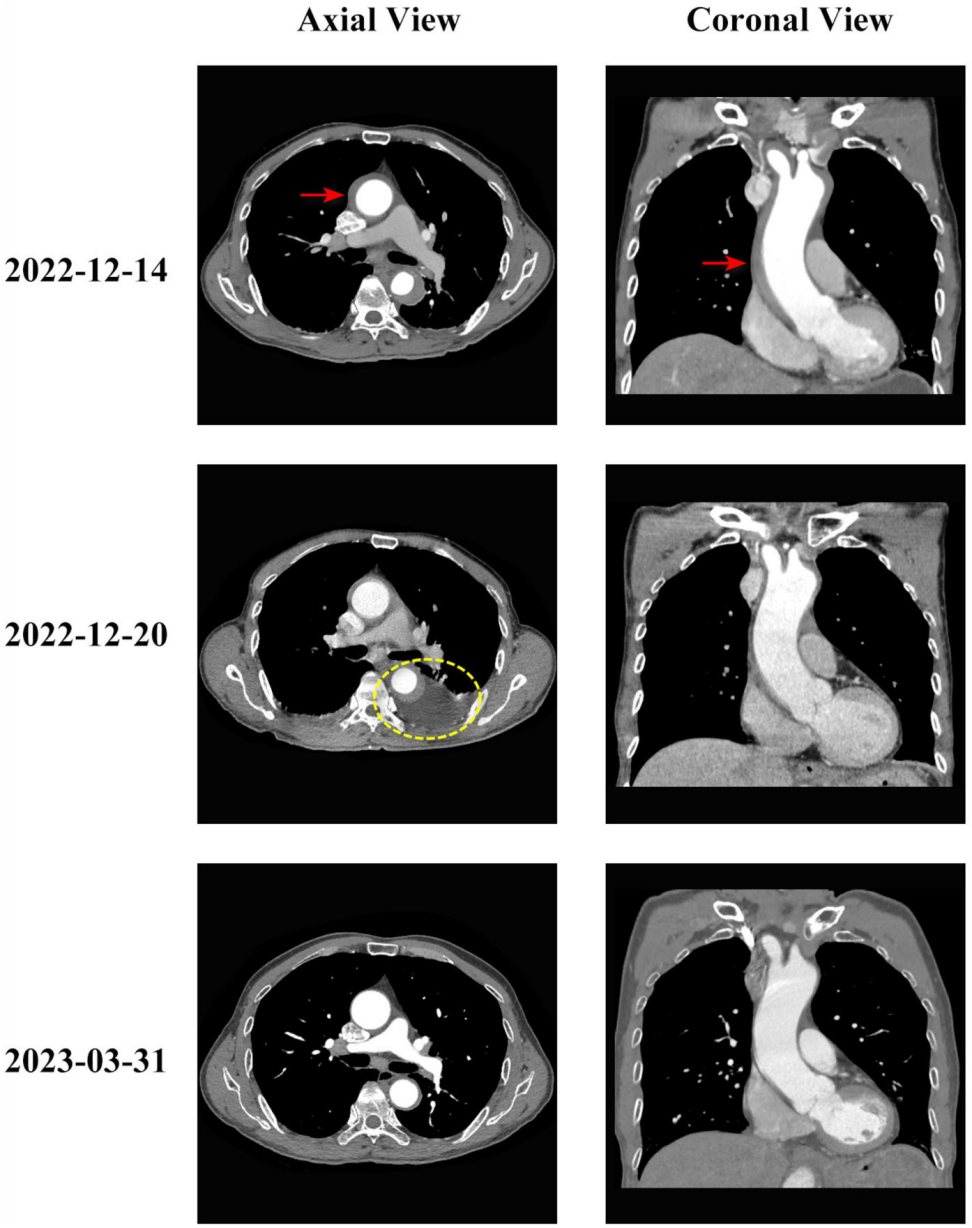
Evolution of type A aortic intramural hematoma during conservative treatment. Serial computed tomography angiography (CTA) images showing the evolution of type A aortic intramural hematoma in axial (left column) and coronal (right column) views. Top row (2022-12-14): Initial presentation showing type A intramural hematoma involving the ascending aorta (red arrows indicate intramural hematoma location) with thickening of the aortic wall (hematoma thickness 7 mm). The ascending aortic diameter measured 42 mm. Middle row (2022-12-20): One weeks after conservative treatment initiation, showing resolution of the ascending aortic intramural hematoma but development of periaortic hematoma around the descending aorta (yellow circle indicates periaortic hematoma). Bottom row (2023-03-31): Three months follow-up demonstrating complete resolution of both the intramural hematoma and periaortic hematoma, with restoration of normal aortic wall appearance. The patient remained asymptomatic throughout the follow-up period.
